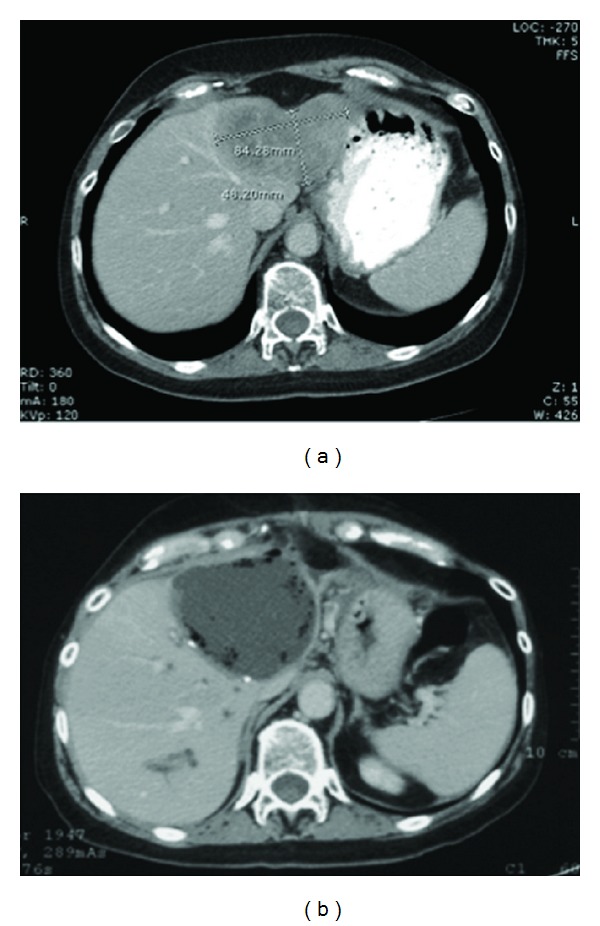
Preoperative and postoperative CT scan of patient. (a) Preoperative CT scan of this patient demonstrating a large metastatic breast cancer tumor (8.4 × 4.6 cm) located in the left hemiliver abutting the middle hepatic vein. An extended left hepatectomy including caudate lobectomy was performed with negative margins. (b) CT scan performed two weeks after hepatectomy demonstrating a large biloma located in the post-hepatectomy bed.
